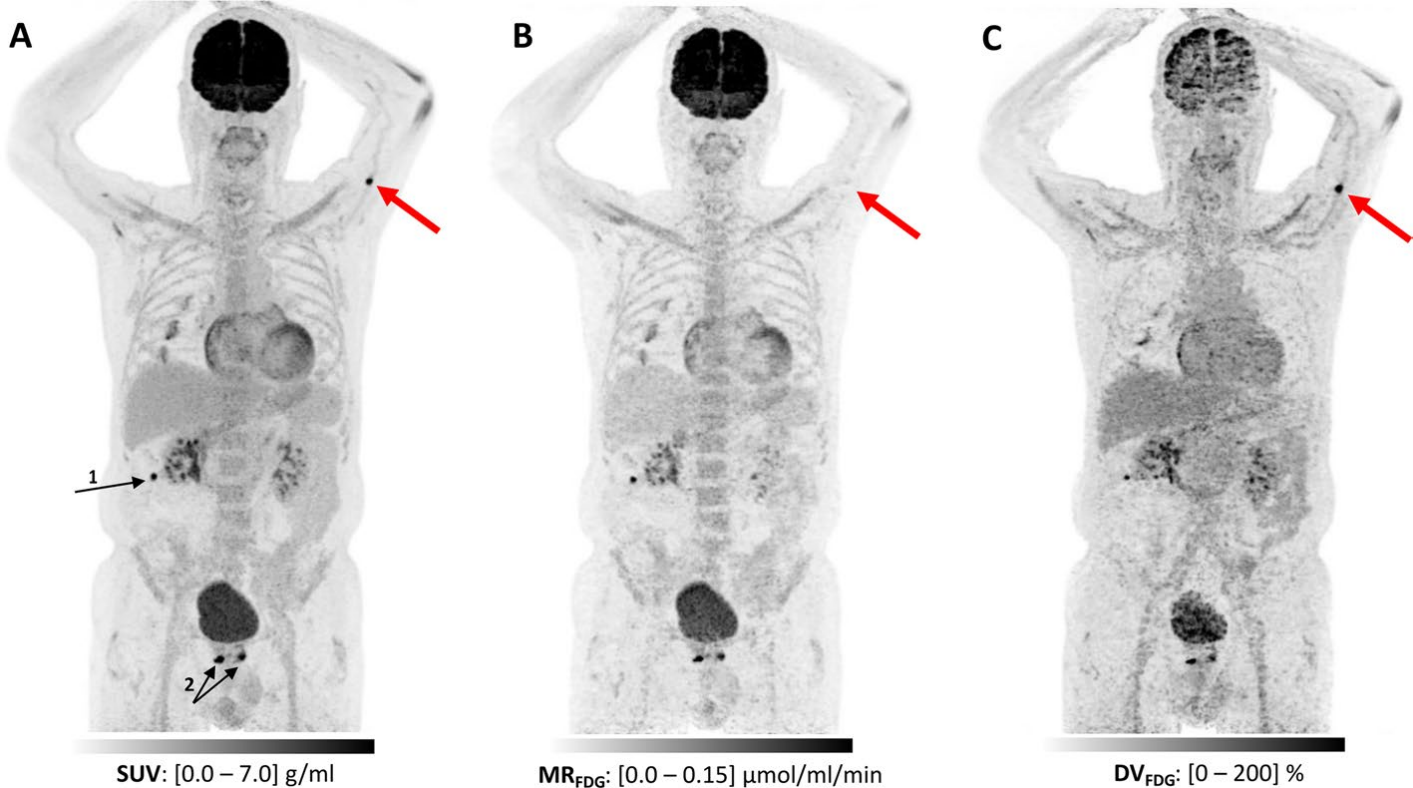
MIPs of the three resulting multiparametric images from a 20-min D-WB examination with four 5 min passes from 50 to 70 min post-injection using PBIF. A SUV calculated as the average of all four passes reflecting the total ^18^FDG signal; B Patlak MR_FDG_ reflecting the metabolic rate of ^18^FDG, i.e. conversion into ^18^FDG-6P; C Patlak DV_FDG_ reflecting the distribution volume of free ^18^FDG. This 85-year-old man was referred for interim evaluation of treatment response after relapse of diffuse large cell lymphoma. SUV images (A), showed regression of nodes on both sides of the diaphragm, but a suspicious newcomer FDG-avid focus was described on the left arm (red arrows), and supplemental localized consolidating radiation treatment was considered. However, further analysis of the Patlak images showed this to be a false-positive finding, as there is no signal on MR_FDG_ images (B), and the presence of signal only on DV_FDG_ images (C) shows this to be unbound FDG. The patient was thus in disease remission and required no supplemental treatment. There were a couple other findings that required investigation. On the right side of the abdomen (black arrow 1) lies a little FDG-avid focus in the colon, in relation to a polyp that on polypectomy showed a high-grade adenoma. There were also bilateral foci in the prostate (black arrows 2). Transrectal ultra-sound could not exclude malignancy, but no biopsy was taken due to the patients' age and comorbidities
